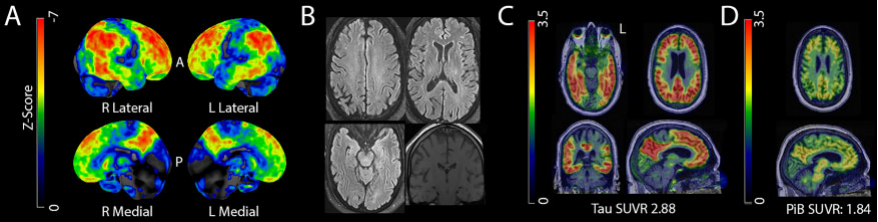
Box 2 Example Case Neuroimaging at presentation (2 years of symptoms) with (A) FDG–PET (Cortex ID, GE Healthcare) showing severe bilateral right > left temporal, parietal, precuneus, posterior cingulate and frontal hypometabolism; (B) MRI Axial T_2_ Flair and Coronal T_1_ images with mild right parietal atrophy with no frontal or hippocampal atrophy; (C) ^18^F-AV1451 tau PET with severe global tau radiotracer uptake; (D) Positive PiB amyloid-PET.
